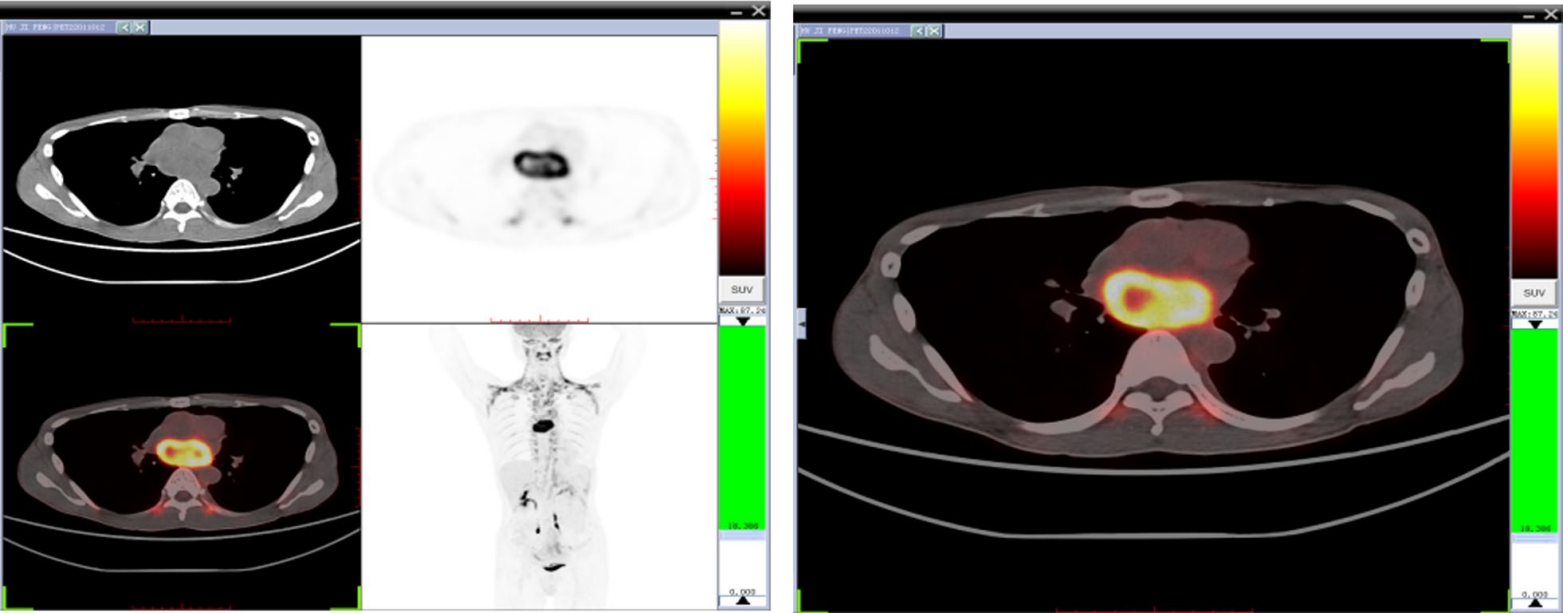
PET-CT The arrow indicates a hypermetabolic mass (3.5 * 6.5 cm) in the left atrium
